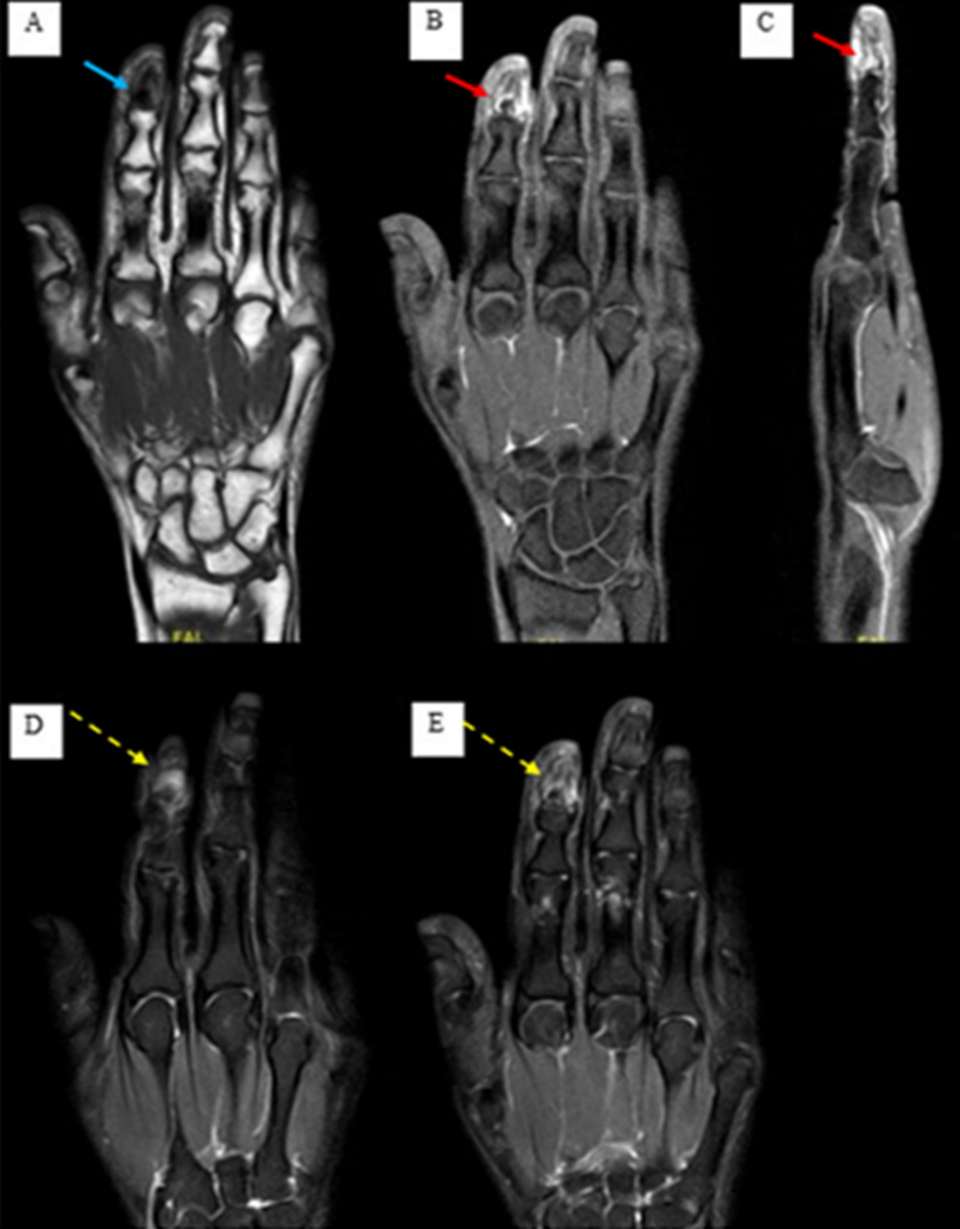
Preoperative MRI of the right hand showing an intramedullary lesion of the distal phalanx of the index finger with infiltration of the bone marrow, cortex, and surrounding tissue with focal sclerosis and elements of enhancement. (A) Hypo-intense on T1-weighted images (blue arrow) showing post-contract enhancement (B and C) (red arrows). Hyper-intense on T2 fat sat weighted images (D and E) (yellow arrows).
